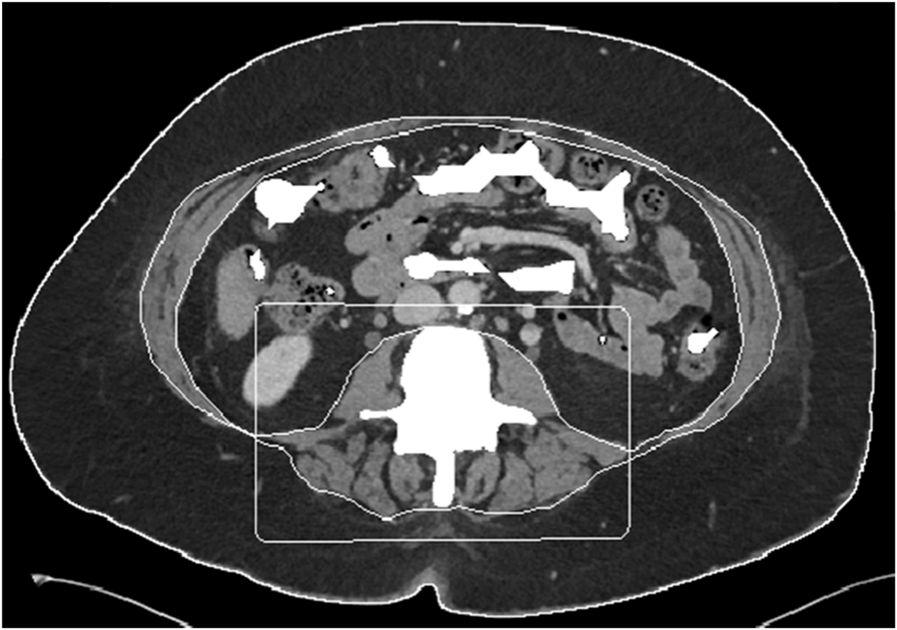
Segmentation of a computed tomography image of a 57-year-old sarcopenic female with a skeletal muscle index of 33 cm^2^/m^2^
